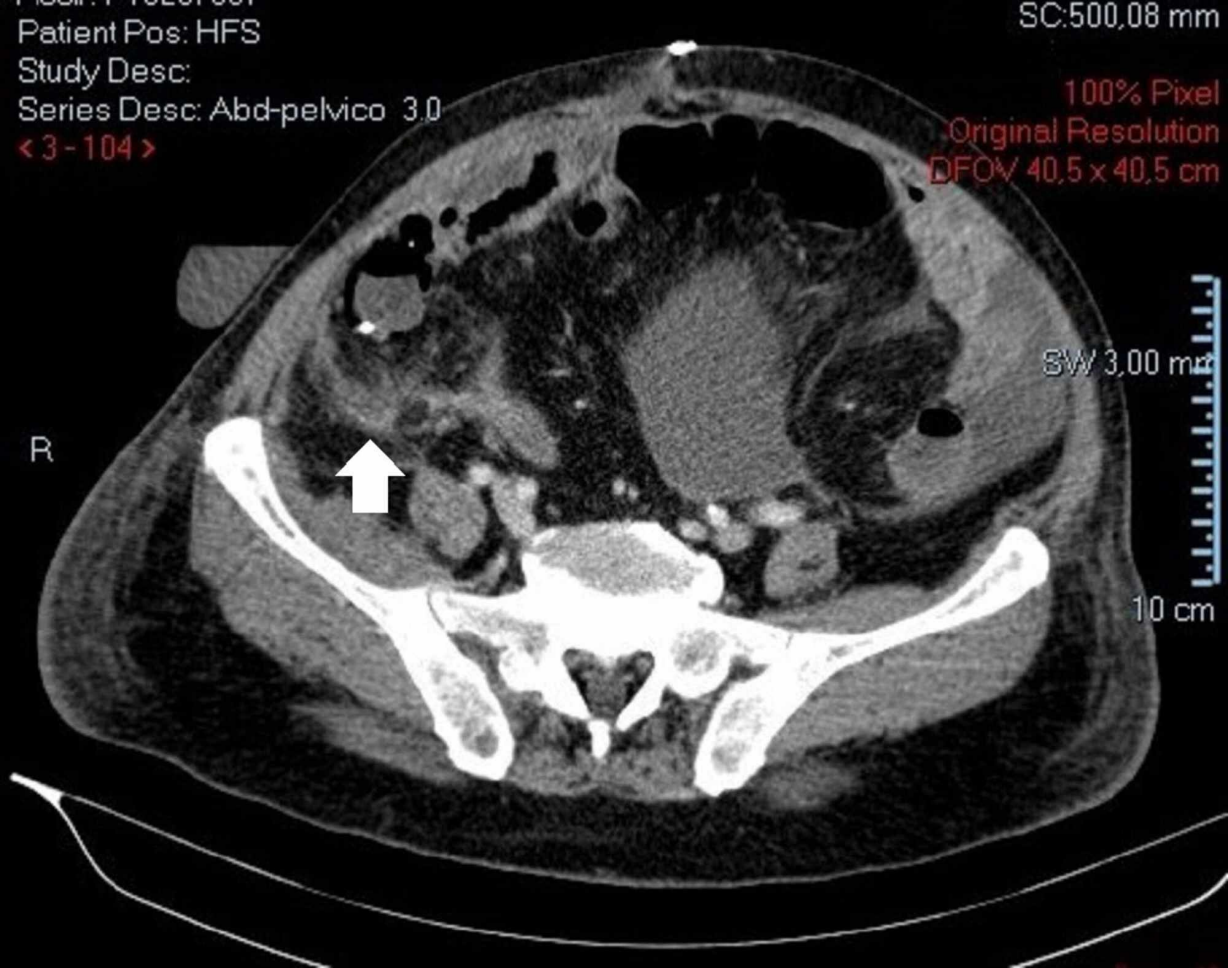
Contrast enhanced abdominopelvic CT scan. Peritoneal thickening with contrast uptake and mesenteric fat densification suggestive of peritonitis.
